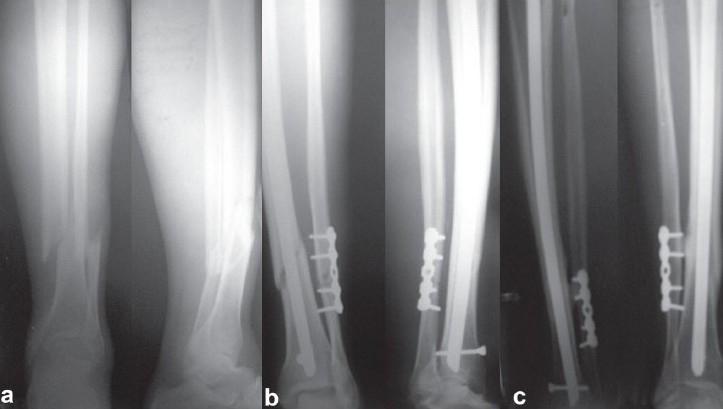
X-ray of left leg (anteroposterior and lateral views) showing a short oblique diaphyseal fracture of distal one fourth of tibia and fibula, which are inherently unstable. (b) Immediate postoperative (anteroposterior and lateral)radiographs showing good fracture reduction. (c) radiograph showing good consolidation at 9 month follow-up.
